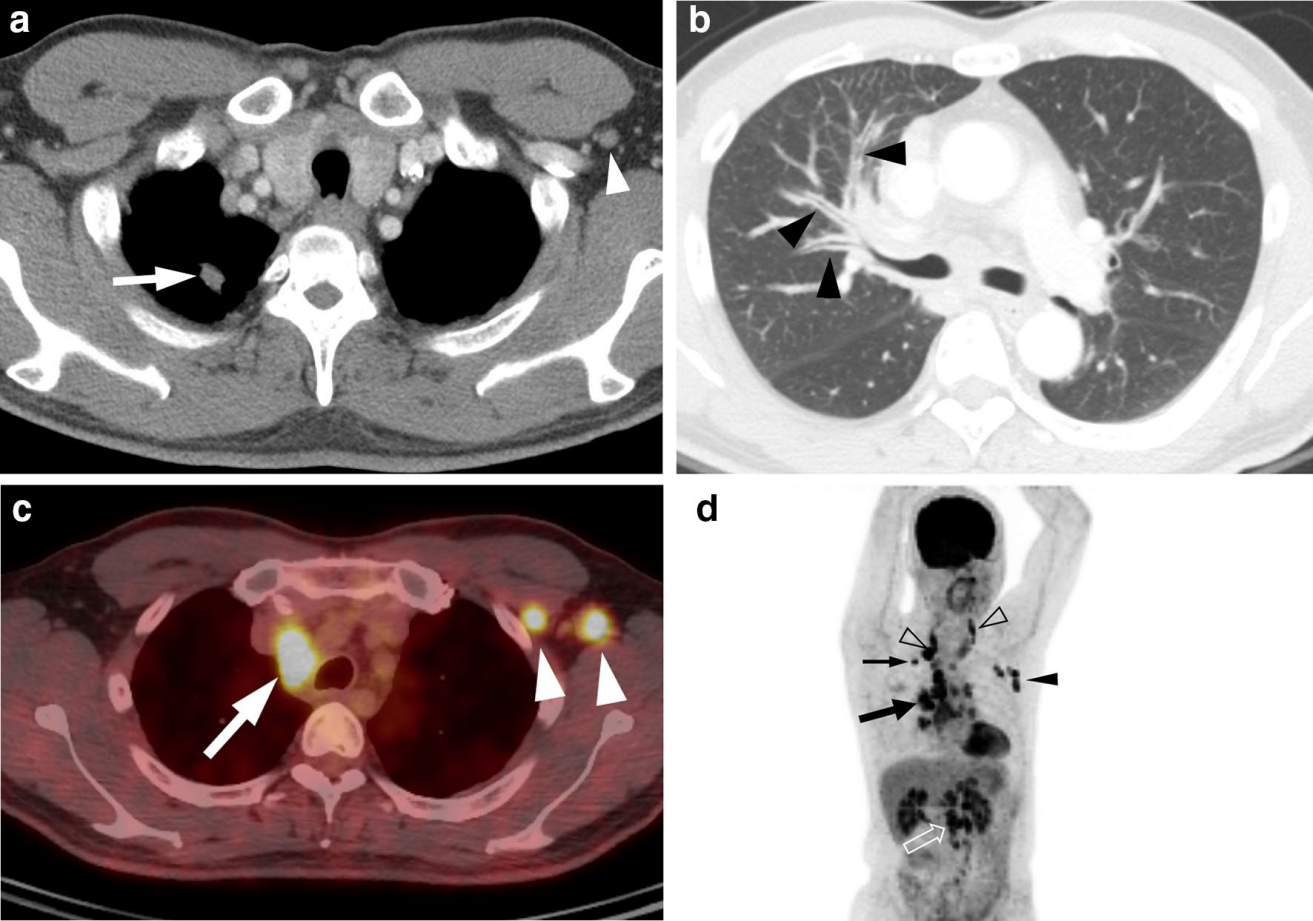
A 44-year-old man with *ROS1*-rearranged lung adenocarcinoma with extensive lymph node metastases. (a) Transverse mediastinal CT image demonstrates a small solid nodule (arrow) in the right upper lobe, which is presumed to be a primary tumor. Left axillary lymph node enlargement (arrowhead) is also noted. (b) Transverse lung window CT image shows diffuse bronchial wall thickening (arrowheads), which represents lymphangitic carcinomatosis. (c) Fused PET/CT image demonstrates fluorodeoxyglucose (FDG)-avid right paratracheal (arrow) and left axillary (arrowheads) lymph nodes. (d) Maximum intensity projection image of PET shows intense FDG uptake in the primary tumor (thin arrow), cervical (open arrowheads), mediastinal (thick arrow), left axillary (arrowhead), and intraabdominal (open arrow) lymph node metastases.
